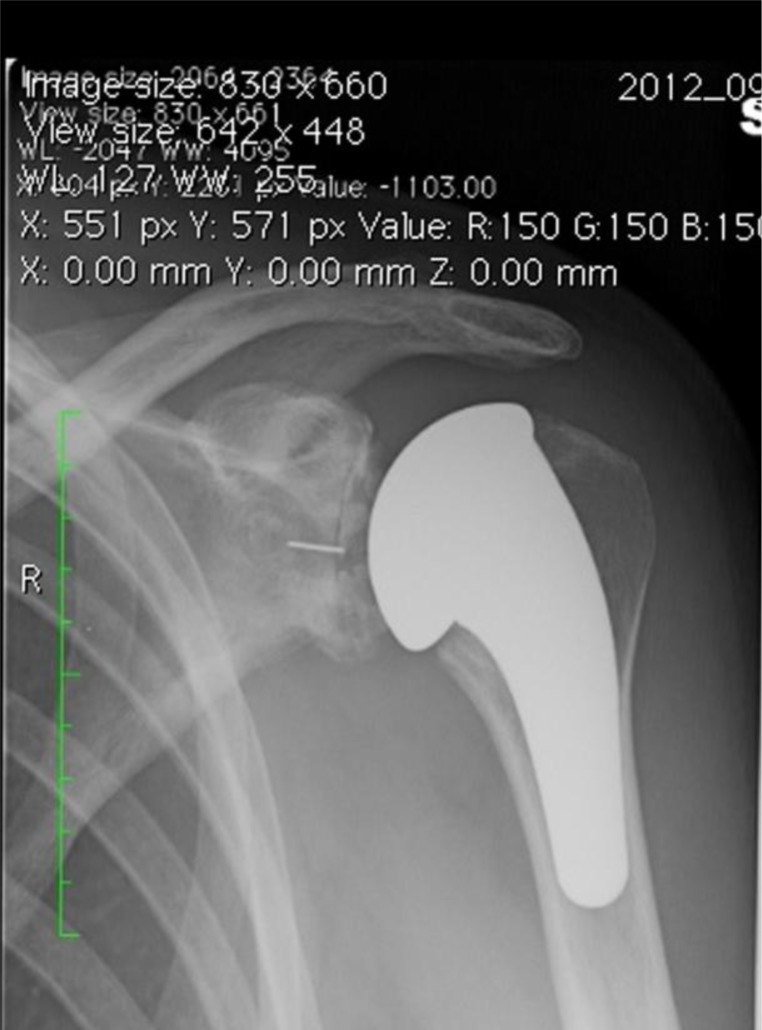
Postoperative X-ray of uncemented short stem TSA (Tornier, Inc, Montbonnot Saint Martin, France).
